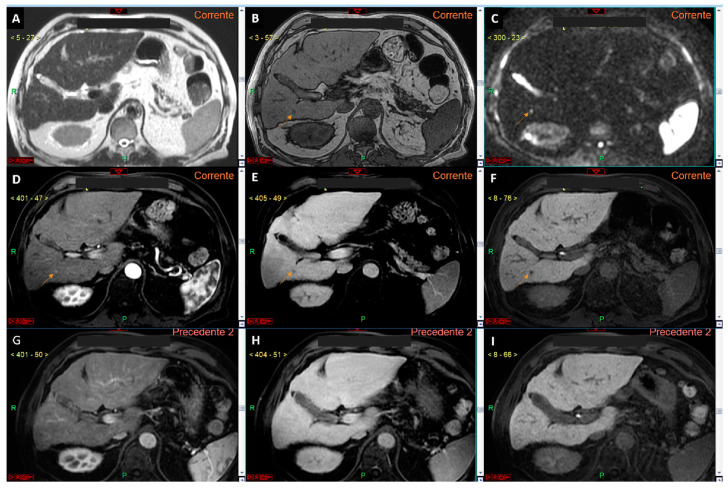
Panels (A–F) represent a complete liver MRI examination with a hepatospecific contrast agent in a cirrhotic liver. Except for the T2-weighted image (A), the remaining images reveal a lesion of 4.5 mm in the liver segment VI (arrows in (B–F)) with features suspicious for HCC. Specifically, the lesion appears hypointense in the T1 out-of-phase sequence (B), shows signal restriction on diffusion-weighted imaging at a b-value 800 (C), arterial phase hyperenhancement (D), wash-out of contrast media in the venous phase (E), and hypointensity in the hepatobiliary phase (F). Panels (G–I) show a study of the same patient performed one year prior to the current examination, where no lesion in the liver segment VI was observed in the arterial (G), venous (H), or hepatobiliary (I) phases. This study demonstrates that, while the segment VI lesion has imaging features typical of HCC, it cannot be diagnosed as such according to EASL guidelines because its size is <1 cm, despite the previous negative MRI exam. However, if the new diagnostic criteria proposed by Huang et al. (see reference no. [25]) were applied, the lesion could be diagnosed as HCC due to an alpha-fetoprotein level of 37 ng/mL.
